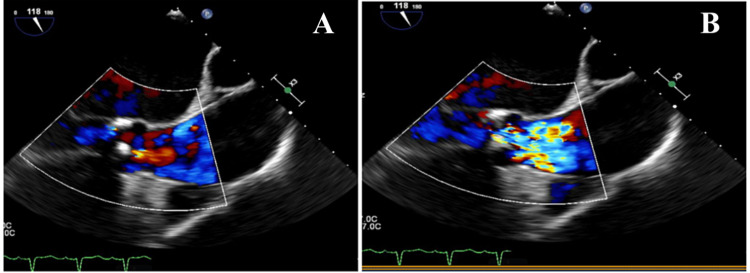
Transthoracic echocardiogram showing aortic regurgitation during diastolic phase (A) and systolic phase (B)
